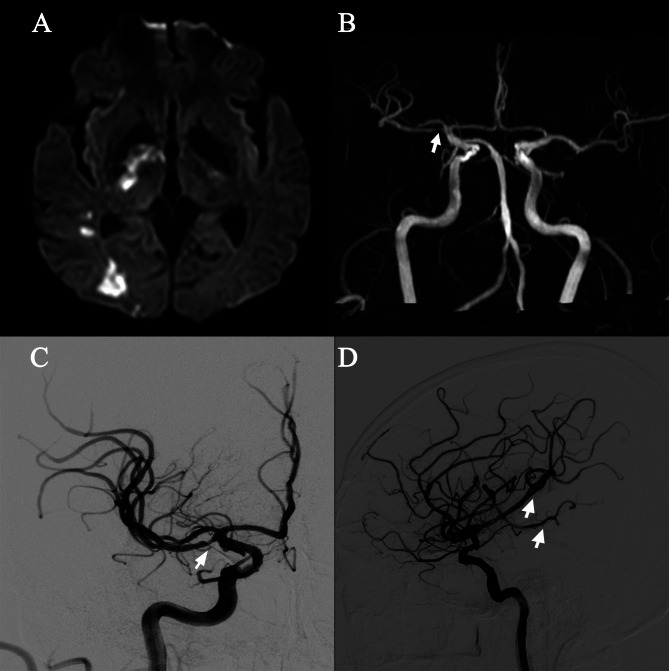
(A) DWI-sequence axial MRI showed multiple fresh infarcts in the right basal ganglia and temporal lobe. (B, C) MRA and DSA revealed that the DMCA (arrow) originated from the distal end of the ICA, with stenosis at the origin. (D) DMCA’s branches in the lateral view of the angiography (arrow)
